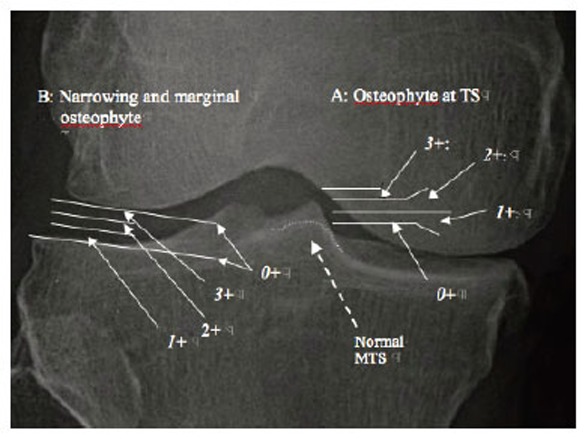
Radiographic OAK-marker in three grades: A: osteophyte at TS; and B: narrowing and marginal osteophyte at compartment. A dotted line at MTS indicates the borderline of normal MTS before developing osteophyte over it.
